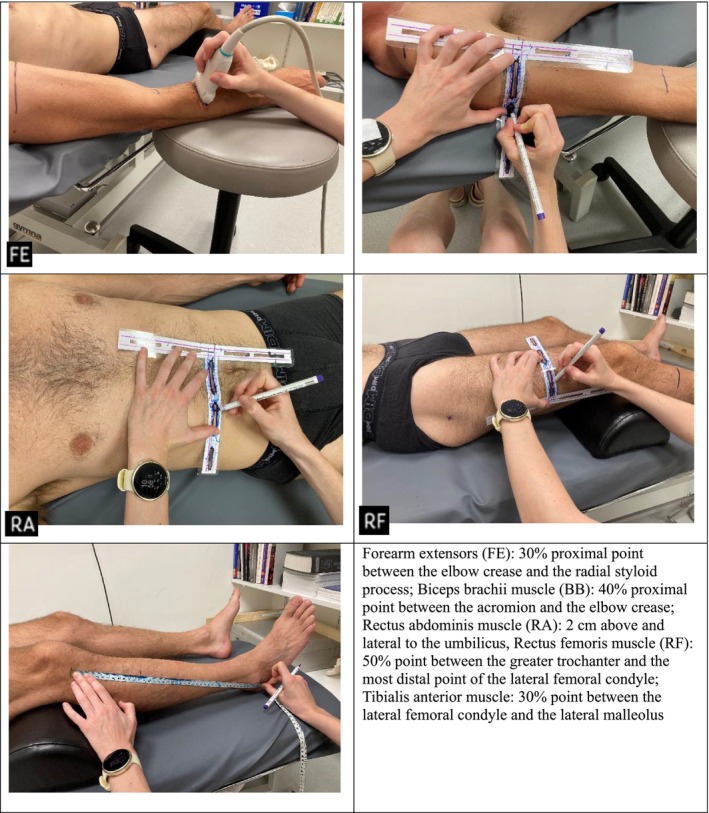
Anatomical landmarks and probe placement sites for ultrasound imaging of the assessed muscles in the supine position.
